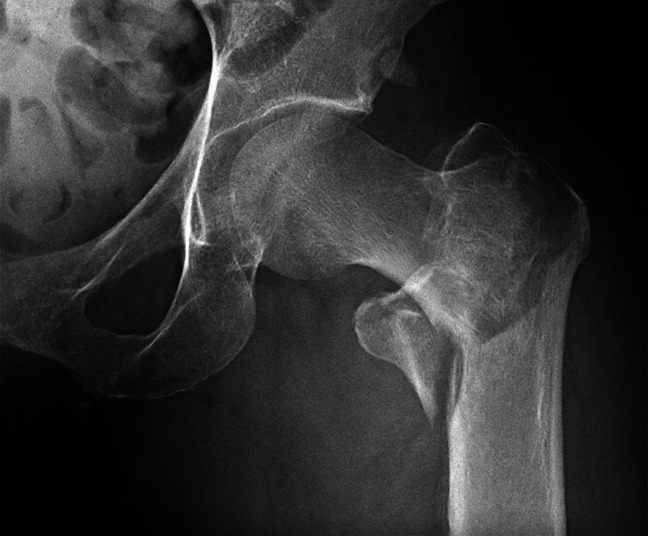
An AP radiograph of the left hip demonstrating an intertrochanteric fracture.
